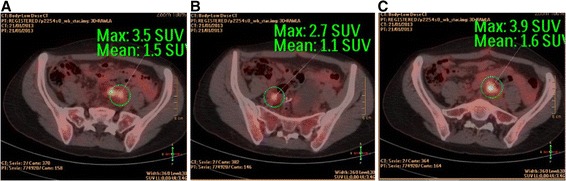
a-c PET-CT pathologic uptake in the pelvis, revealing peritoneal carcinomatosis of colorectal cancer
